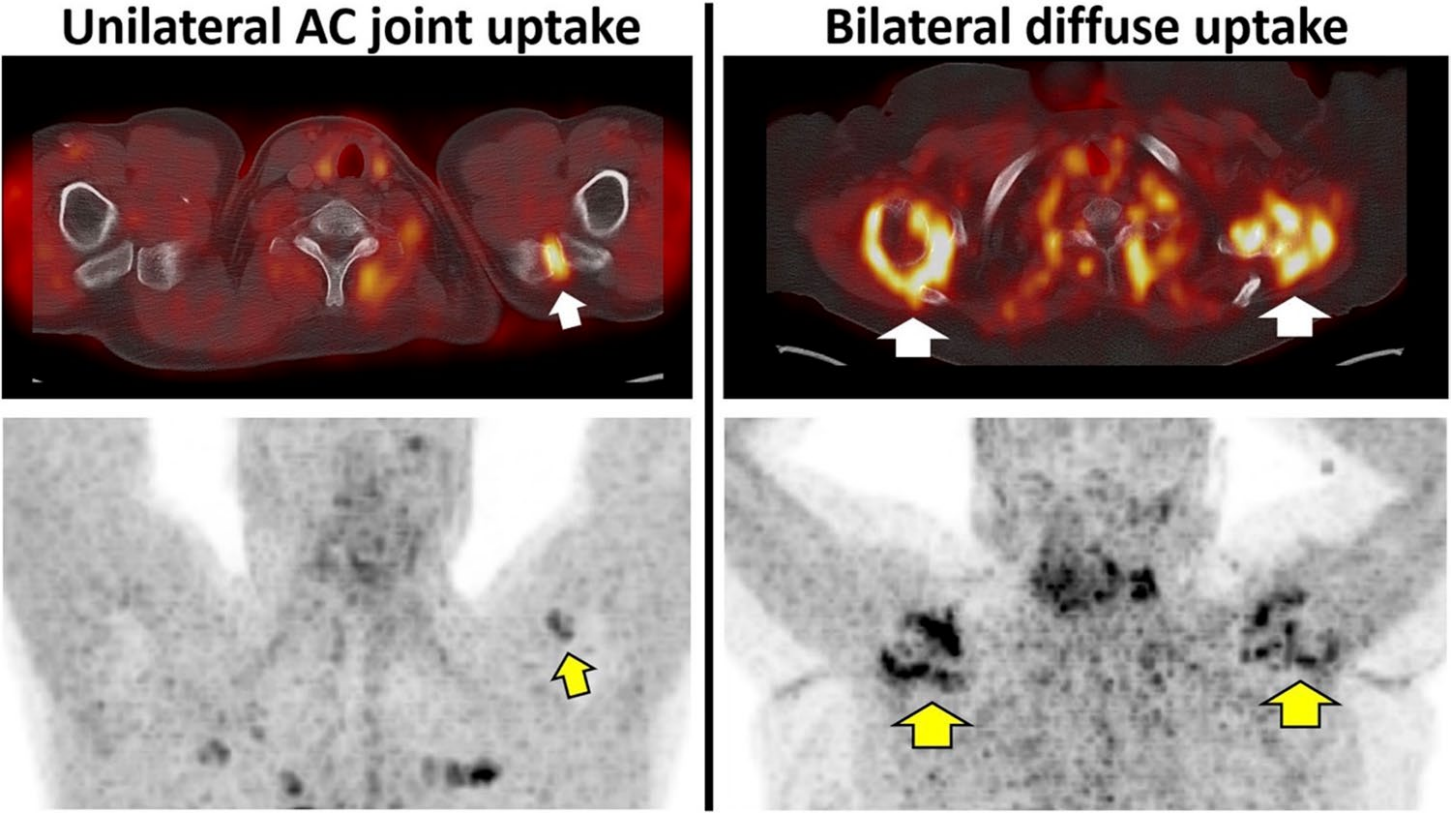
Representative images of Ga-68 FAPI-04 PET. Upper, PET/CT fused images; Lower, PET maximum intensity projection (MIP) images. Arrows indicate intense FAPI uptake in the shoulders. Left, unilateral acromioclavicular joint (AC) uptake of 37-year-old man; Right, bilateral diffuse uptake including uptake in the AC and the glenohumeral plus subacromial joints of 54-year-old woman
